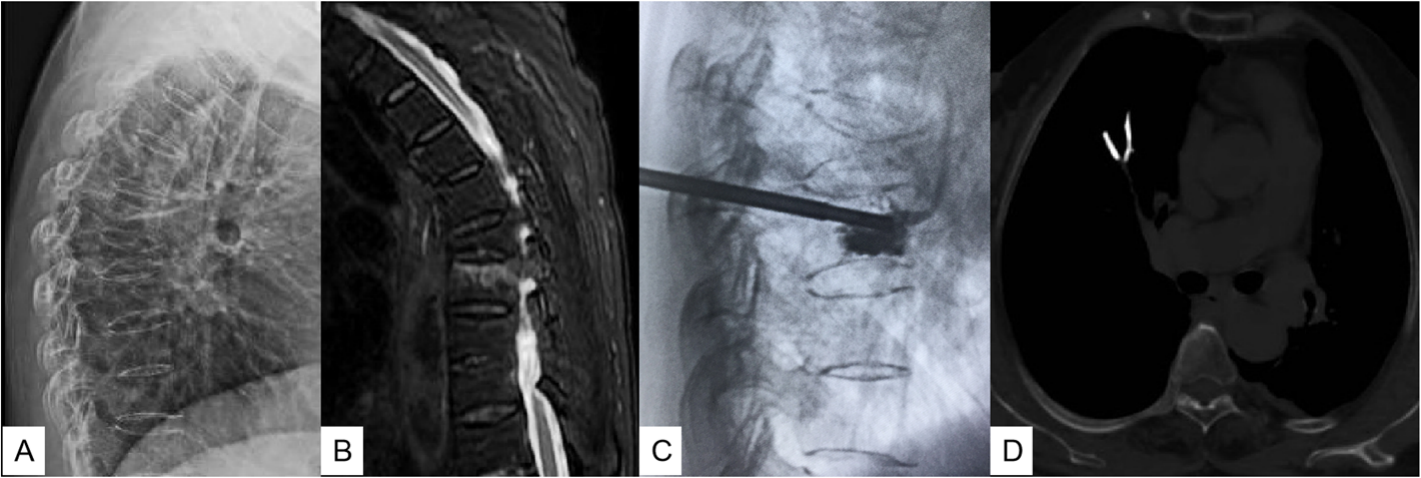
A 72-year-old woman was hospitalized complaining of severe back pain after falling down 2 weeks before admission. Preoperative thoracic lateral X-ray showed T7 vertebral compression fracture (a). Sagittal MRI (fat-suppressed T2-weighted images) showed that T7 was old fracture, while T8 was new osteoporotic compression fracture (b). Intraoperative thoracic lateral fluoroscopy showed that bone cement spilled into the anterior paravertebral vessels (c). Postoperative pulmonary CT found the pulmonary cement emboli of right inferior lung lobe (d)
